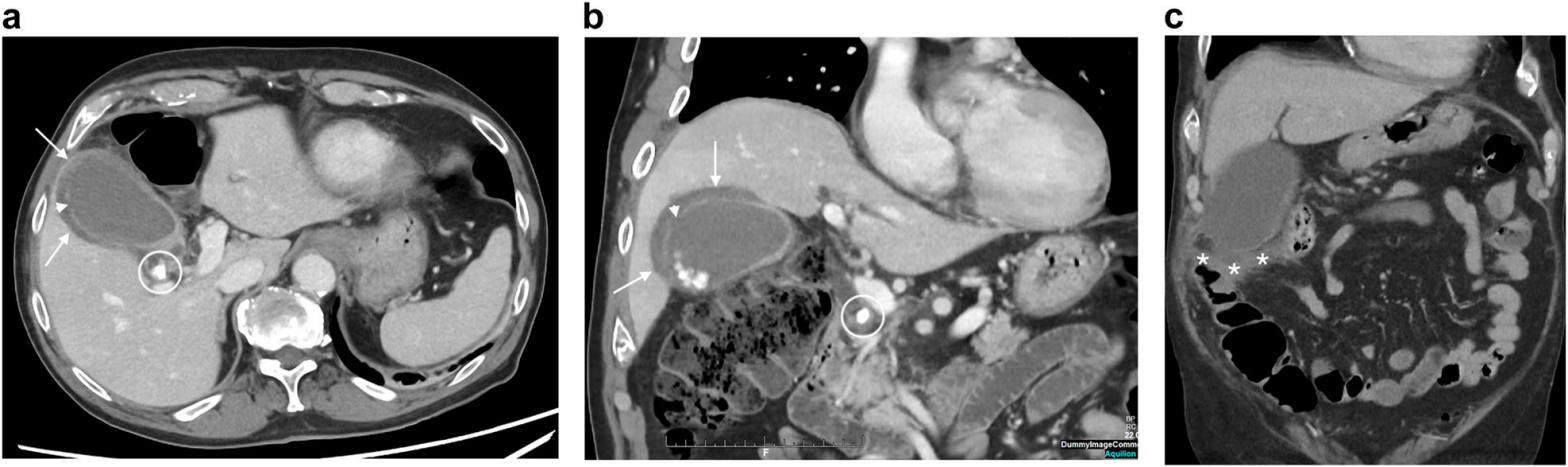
Gangrenous cholecystitis. Axial and coronal portal phase CT (a, b) in an 80-year-old man shows an irregular thickening of the gallbladder wall (arrows) with interrupted contrast enhancement (arrowhead). The cystic duct is obstructed by a gallstone (circle). Coronal portal phase CT (c) in an 81-year-old man shows increased density of fatty tissue around the gallbladder (asterisk) and interruption of the inferior part of the gallbladder wall
